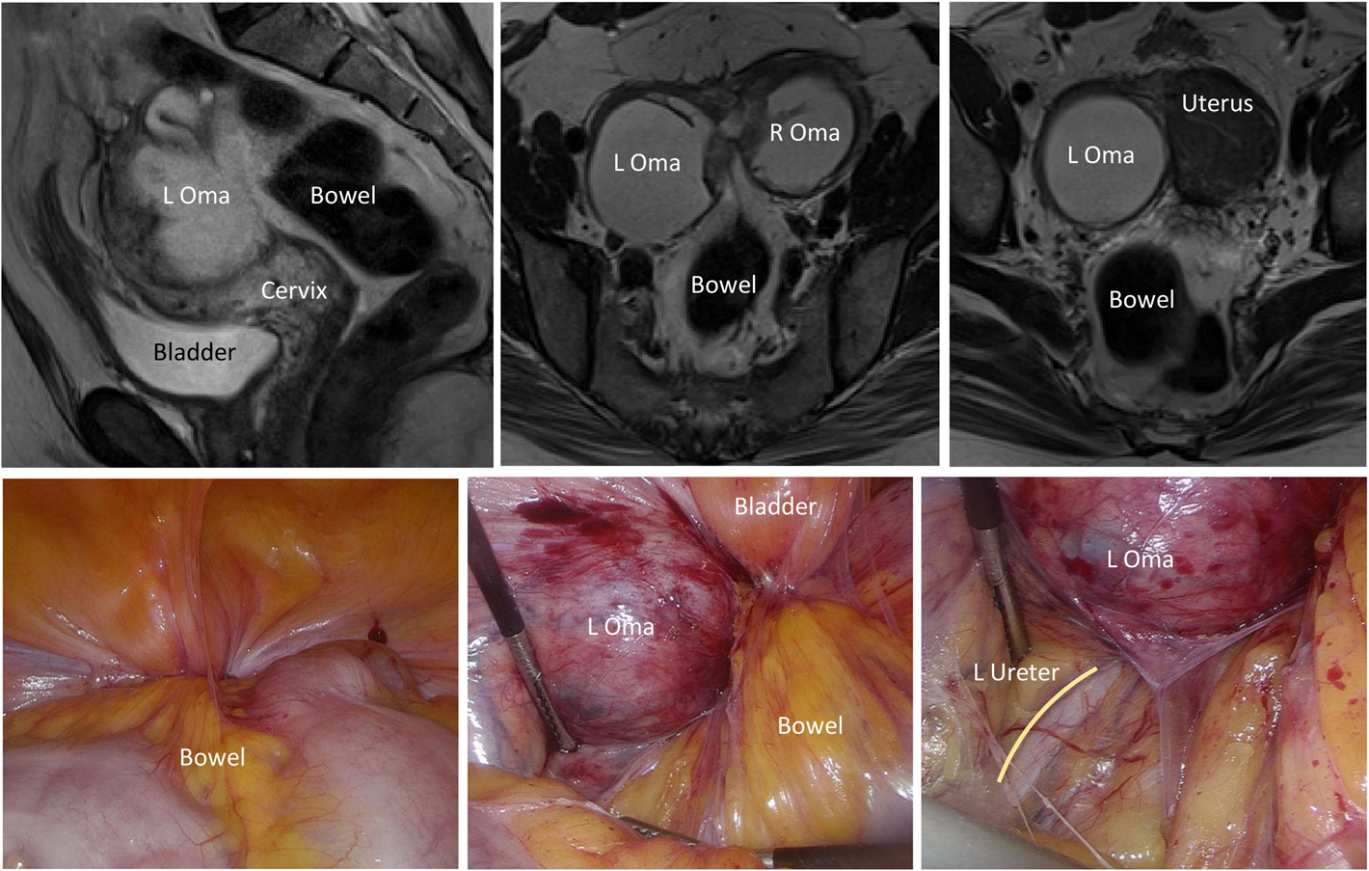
Clinical photos correlating to model in Fig. 4. Sagittal view (top left) and axial views (top middle and top right) of MRI T2 images depicting bilateral endometriomas, distorted bladder, and retroflexion of the uterus’ fundal region. Surgical presentation with no pelvic anatomy seen on initial inspection due to severity of adhesions of the bowel and bladder (bottom left), the bowel adhering to the left endometrioma and bladder (bottom middle), and the close proximity of the left ureter to the left endometrioma (bottom right)
